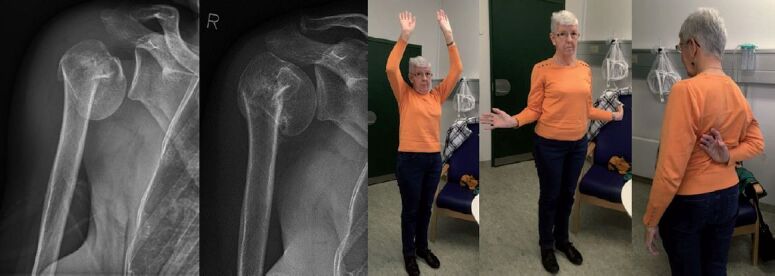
68-year-old female suffering an impacted 2-part fracture of the proximal humerus. Radiographs were taken on admission and after 5 months. Clinical photos were taken after 5 months. Pain-free shoulder function was obtained after 3 months. In this case intuition will tell most orthopedic surgeons to restore the anatomy, most likely by open reduction and internal fixation with a locking plate. Best evidence from randomized trials does not support this decision (Rangan et al. 2015, Launonen et al. 2019). How to act?
